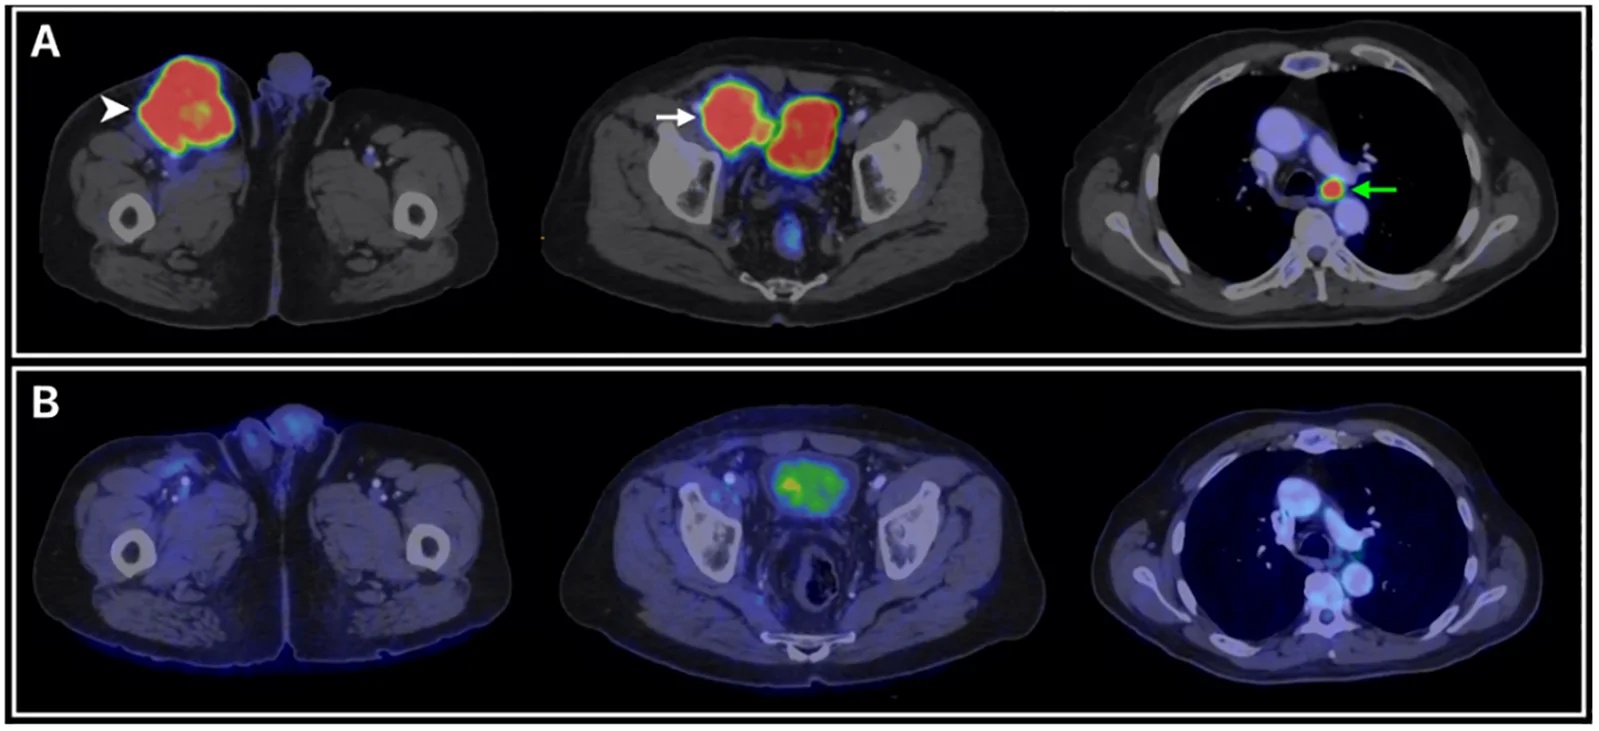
FDG PET-CT at baseline tumor activity and complete response to treatment. (A) Hypermetabolic solid mass in the right thigh (arrowhead), hypermetabolic lymph nodes conglomerate along the right external iliac chain (white arrow), hypermetabolic paratracheal lymph node (green arrow). (B) Complete metabolic and morphologic resolution of all lesions.
